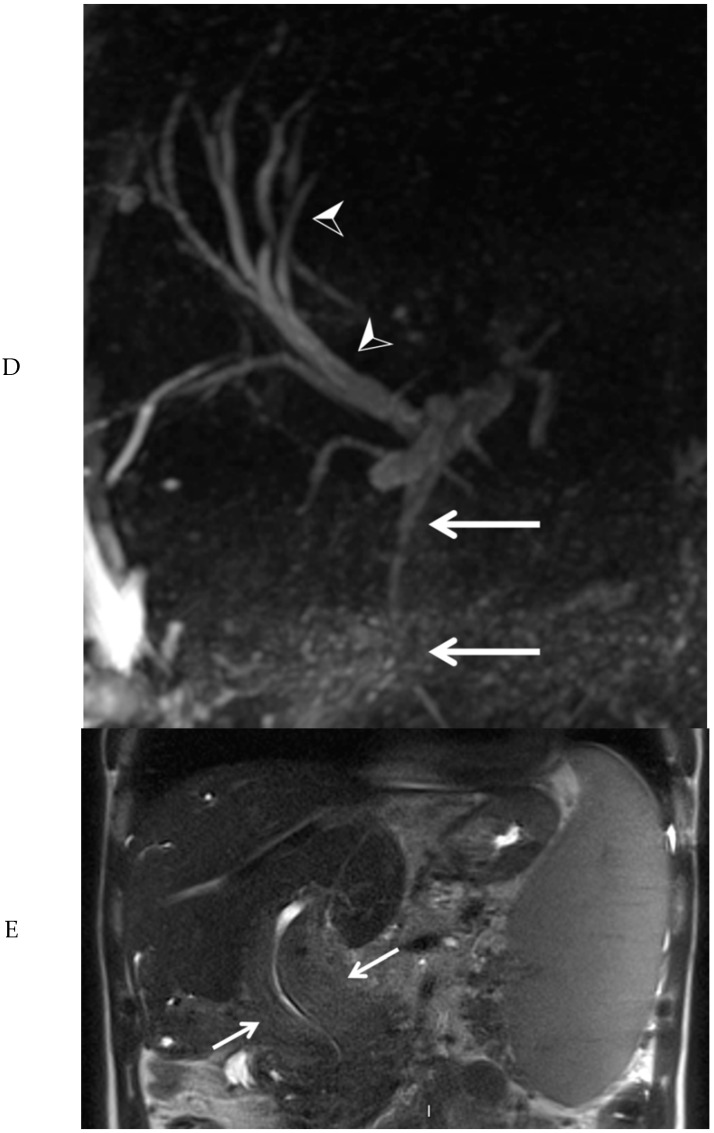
Magnetic resonance imaging of the mass in the upper abdomen (arrows). Shown are T1 weighted subtraction images of the mass in the upper abdomen with fat suppression after administration of intravenous contrast medium in the arterial (A), portal venous (B), and delayed phase (C). At magnetic resonance cholangiopancreaticography, narrowing of the common bile duct is visible along its complete course (arrows), combined with dilation of the intrahepatic bile ducts (arrowheads) (D). At T2-weighted imaging (E, coronal view), the mass surrounding the narrowed common bile duct was slightly hyperintense, when compared to the liver parenchyma (arrows).
